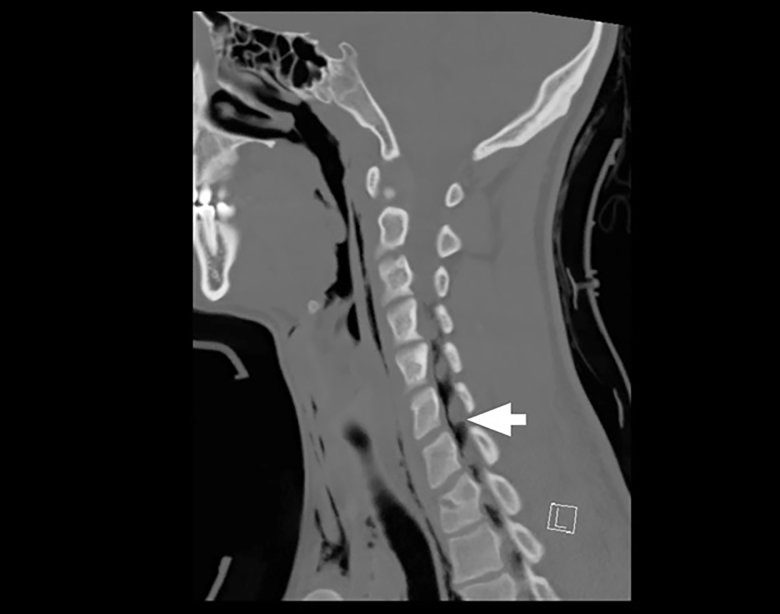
Sagittal view of the patient’s neck computed tomography demonstrates pneumorrhachis (arrow).
